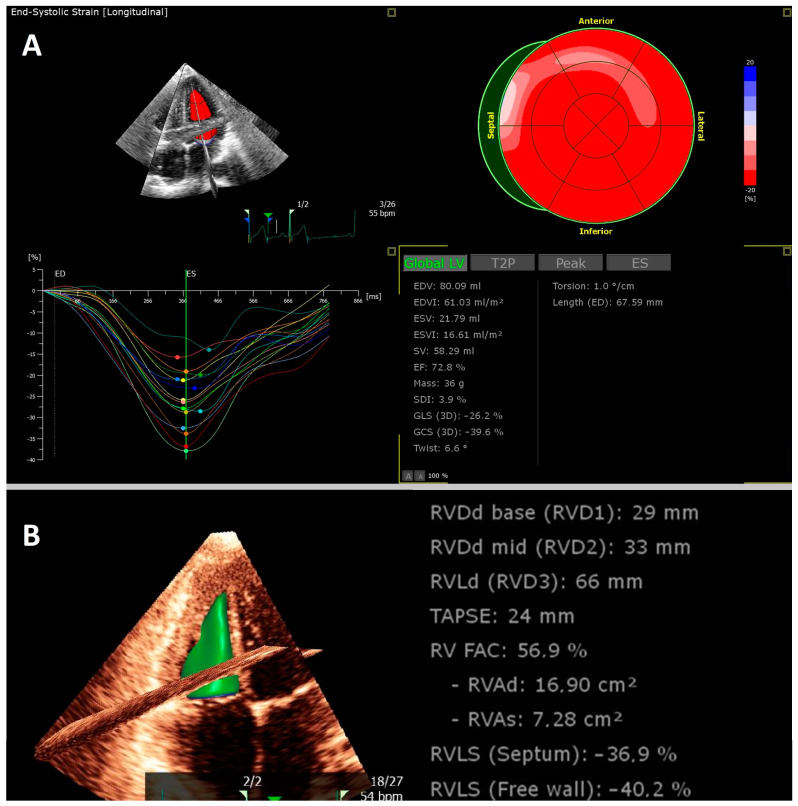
Example of a young athlete who was examined after COVID-19 before returning to sport. Transthoracic 3D echocardiography revealed normal left ventricular (A) and right ventricular function (B).
